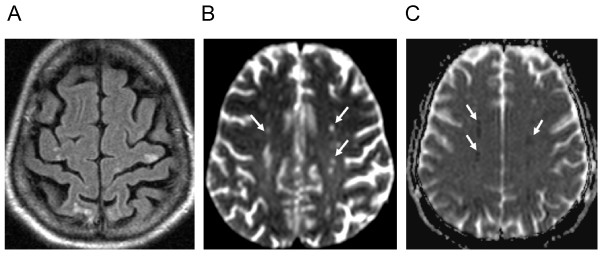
A: axial FLAIR image shows multifocal cortical and subcortical hyperintense lesions (arrows) presumed to be of embolic origin. B and 2 C: axial diffusion-weighted image-DWI (b-value, 1,000 s/mm^2^) (Figure 2B) and corresponding apparent diffusion coefficient (ADC) map demonstrate marked water diffusion restriction in acute embolic ischemia (arrows). Note the drop in signal intensity on ADC-map (Figure 2C, arrows).
